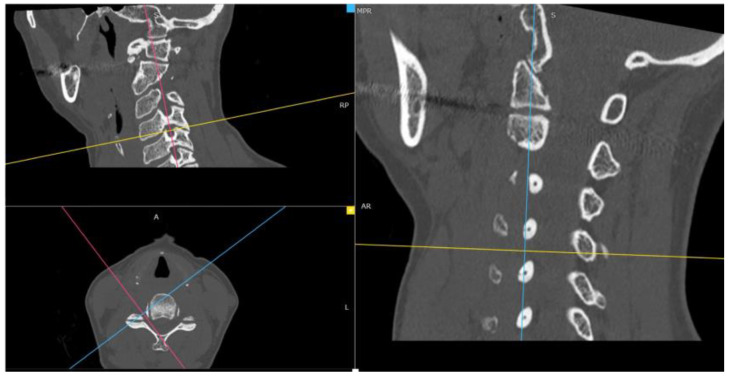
Neural cervical foramen was centered using MIP and MPR CT reconstructions.
